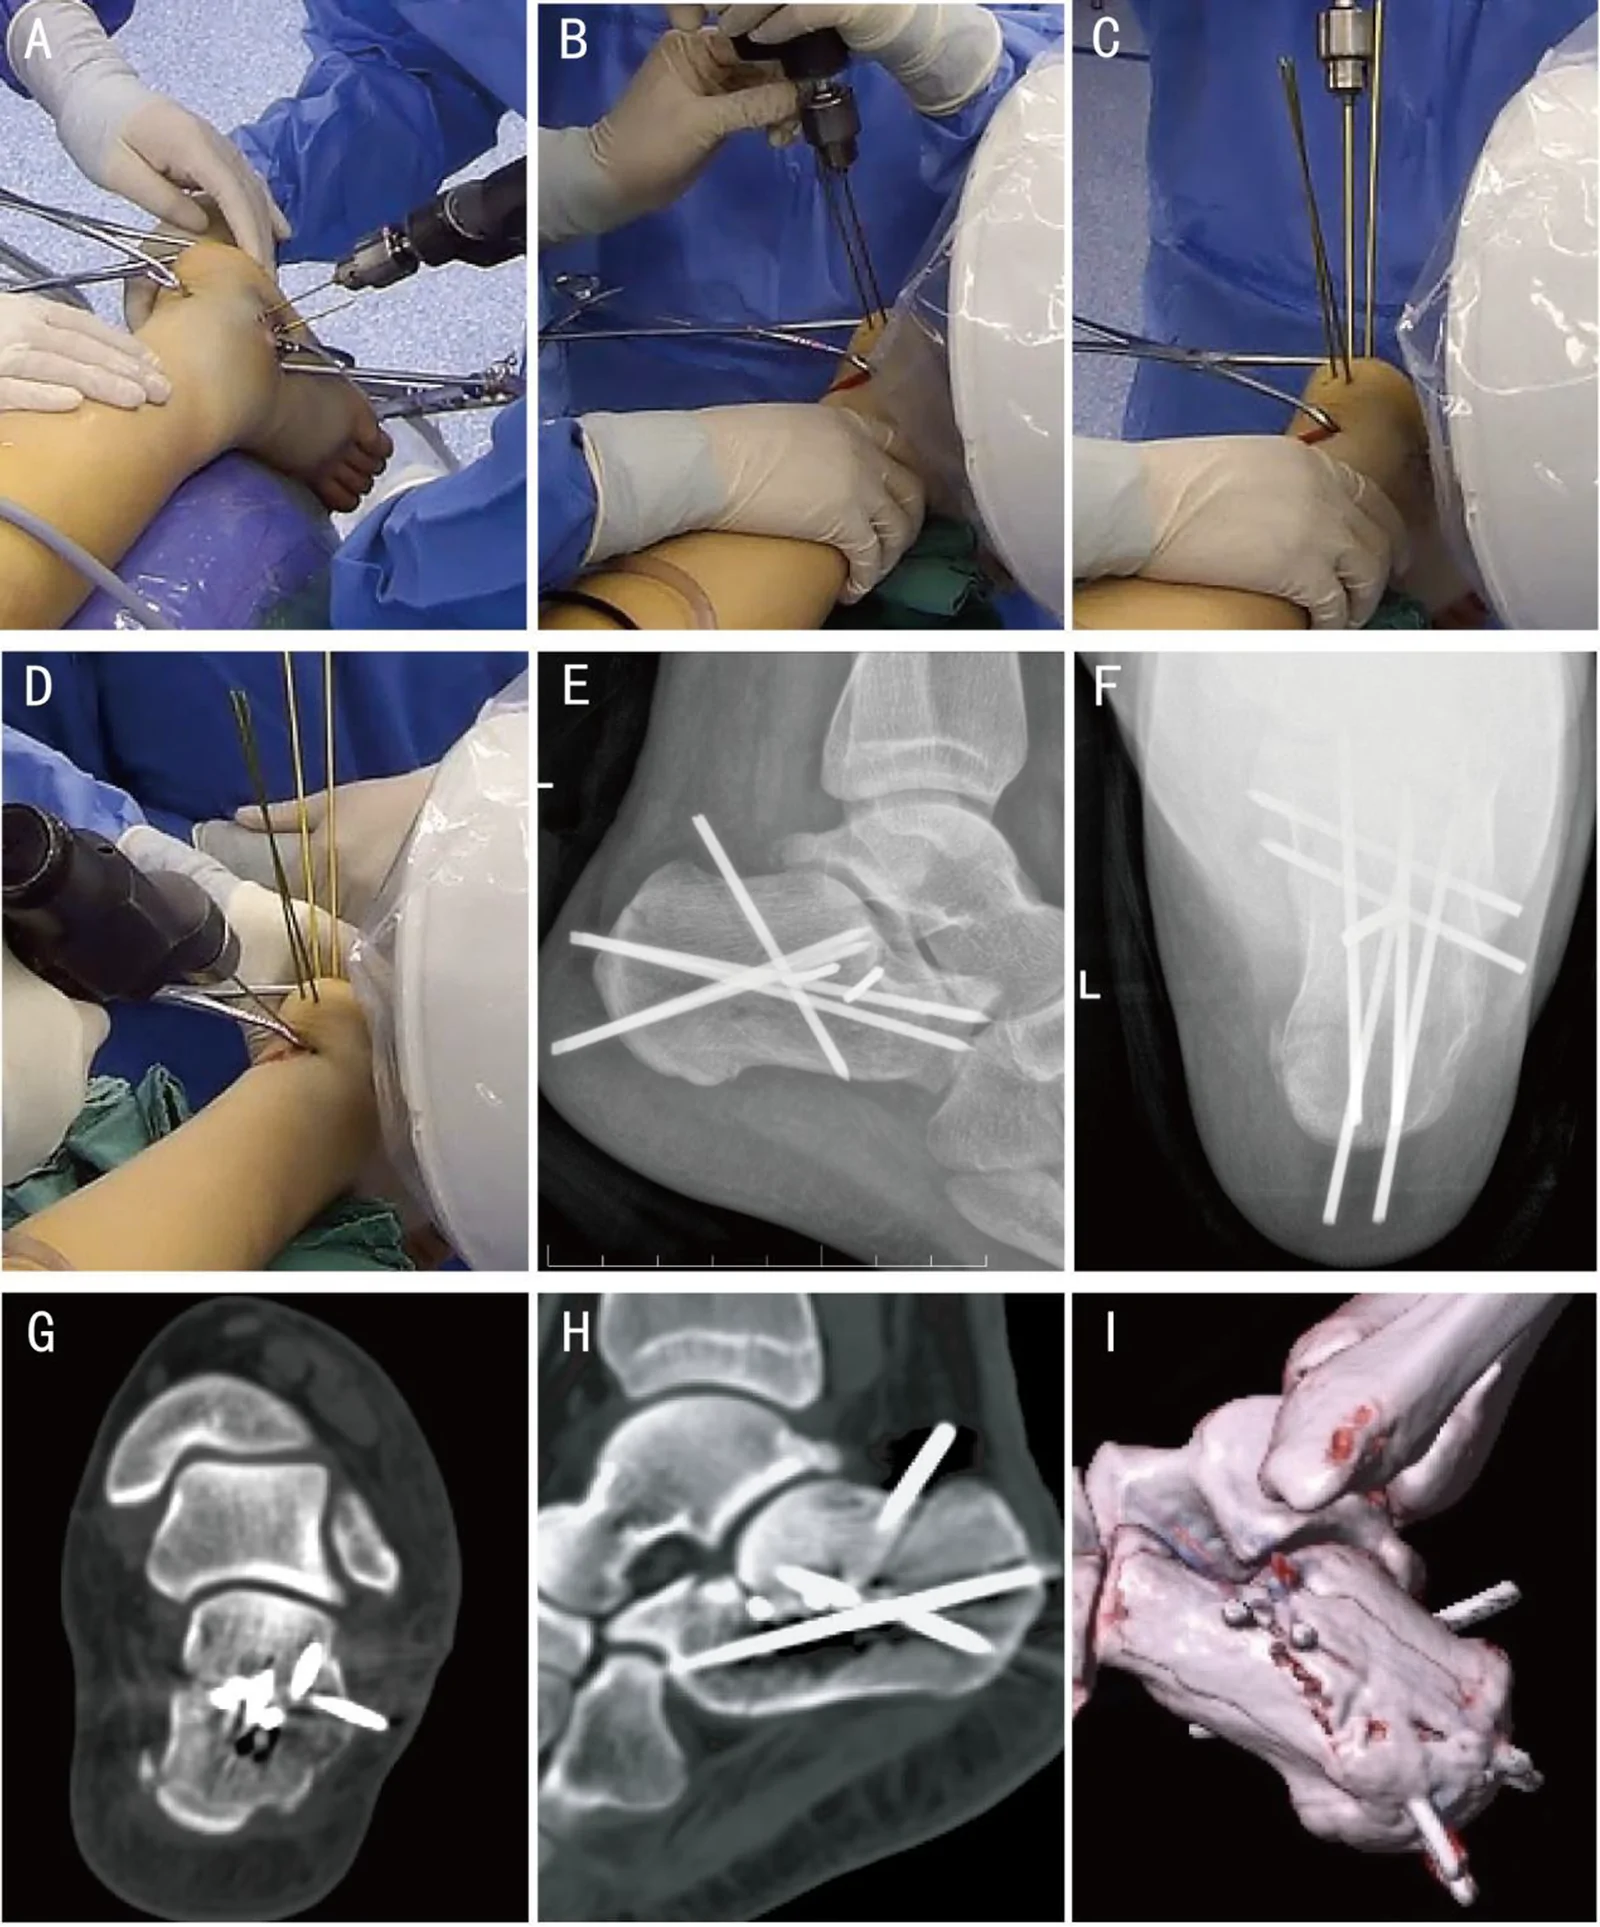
Intraoperative images of percutaneous Kirschner wire fixation: (A) Two 1.5-mm Kirschner wires inserted vertically below the posterior facet on the lateral wall; (B) Two 2.0-mm Kirschner wires driven from the posterior calcaneal tuberosity toward the calcaneocuboid joint; (C) Two 2.0-mm Kirschner wires placed from the plantar side of the tuberosity toward the posterior facet; (D) One 2.0-mm Kirschner wire inserted from the dorsal side of the tuberosity in an anteroinferior direction. Postoperative radiographs of the left calcaneus: (E) Lateral view; (F) Axial view. Postoperative CT images with 3D reconstruction of the left foot: (G) Coronal plane; (H) Sagittal plane; (I) 3D reconstruction.
